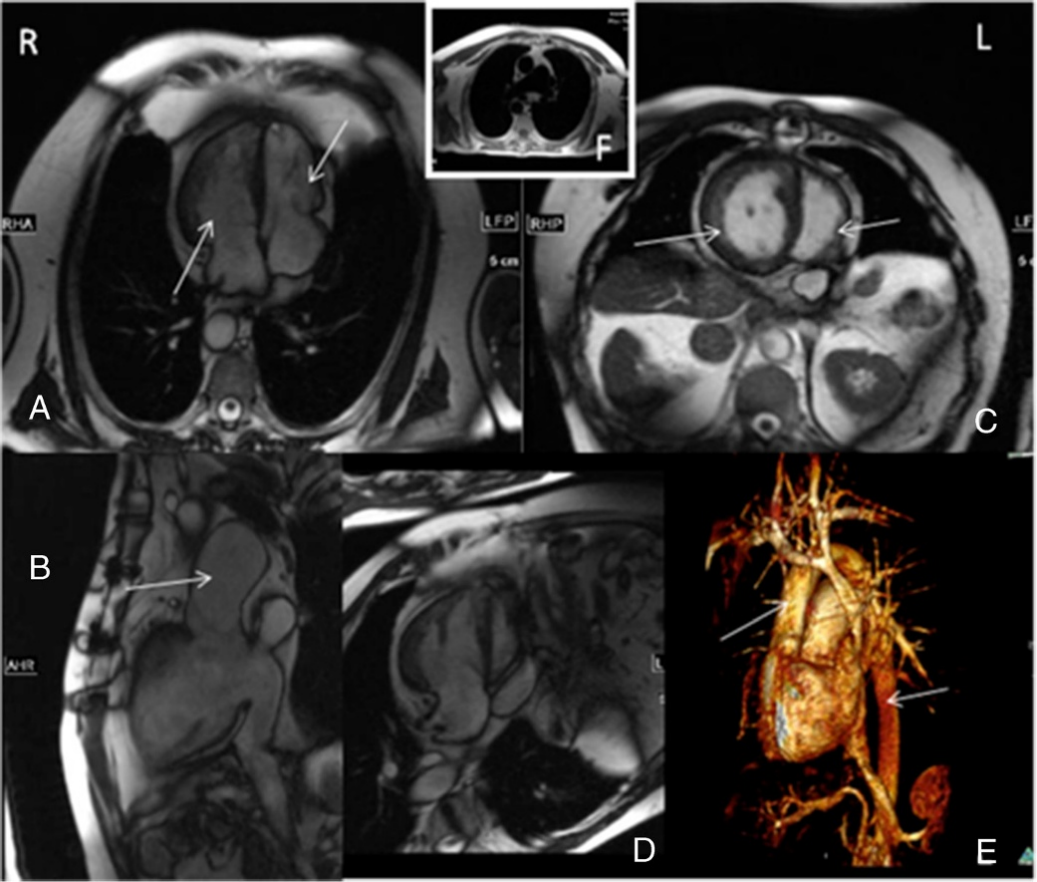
Situs inversus in CMRI. 1.5 T MRI (Magnetom Symphony, Siemens, Healthcare sector, Erlangen, Germany) after application of contrast medium. A: Four-chamber-view with a morphologic right and systemic ventricle (with hypertrophic trabecular structures) as well as a small smoother sub-pulmonary ventricle on the left side (white arrows). B: A dilated pulmonary artery (white arrow) is detected (A). C: Short axis or two-chamber-view (white arrows) demonstrating the cardiac ventricular anatomy of the two ventricles. A D-shaped septum bulging from the systemic right ventricle towards the morphological left ventricle connecting to the pulmonary circulation can be appreciated. D: The outflow of the aortic arch is shown in this image, coming from the functional left ventricle, morphological (original) right ventricle. E: The three-dimensional reconstruction shows the left-sided aorta and right-sided pulmonary trunk (white arrows). F: This image demonstrates the dilated pulmonary trunk and proximal pulmonary arteries in the initial Haste-sequences.
